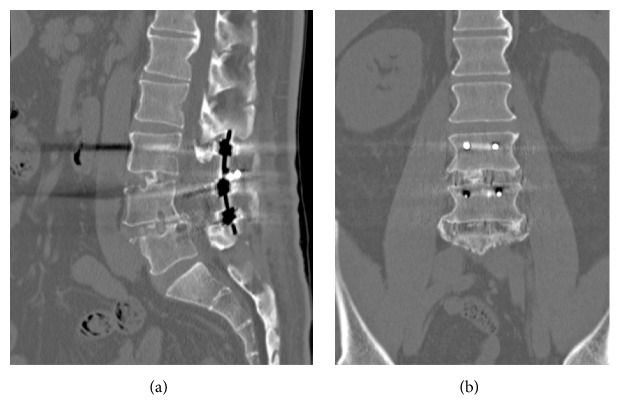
CT 12 months after surgery. Sagittal image at the midspinal canal (a); coronal image at the midvertebral body (b). Bridging bone formation is present at the edge of the cage inserted at the L3/4 and L4/5 intervertebral spaces.
